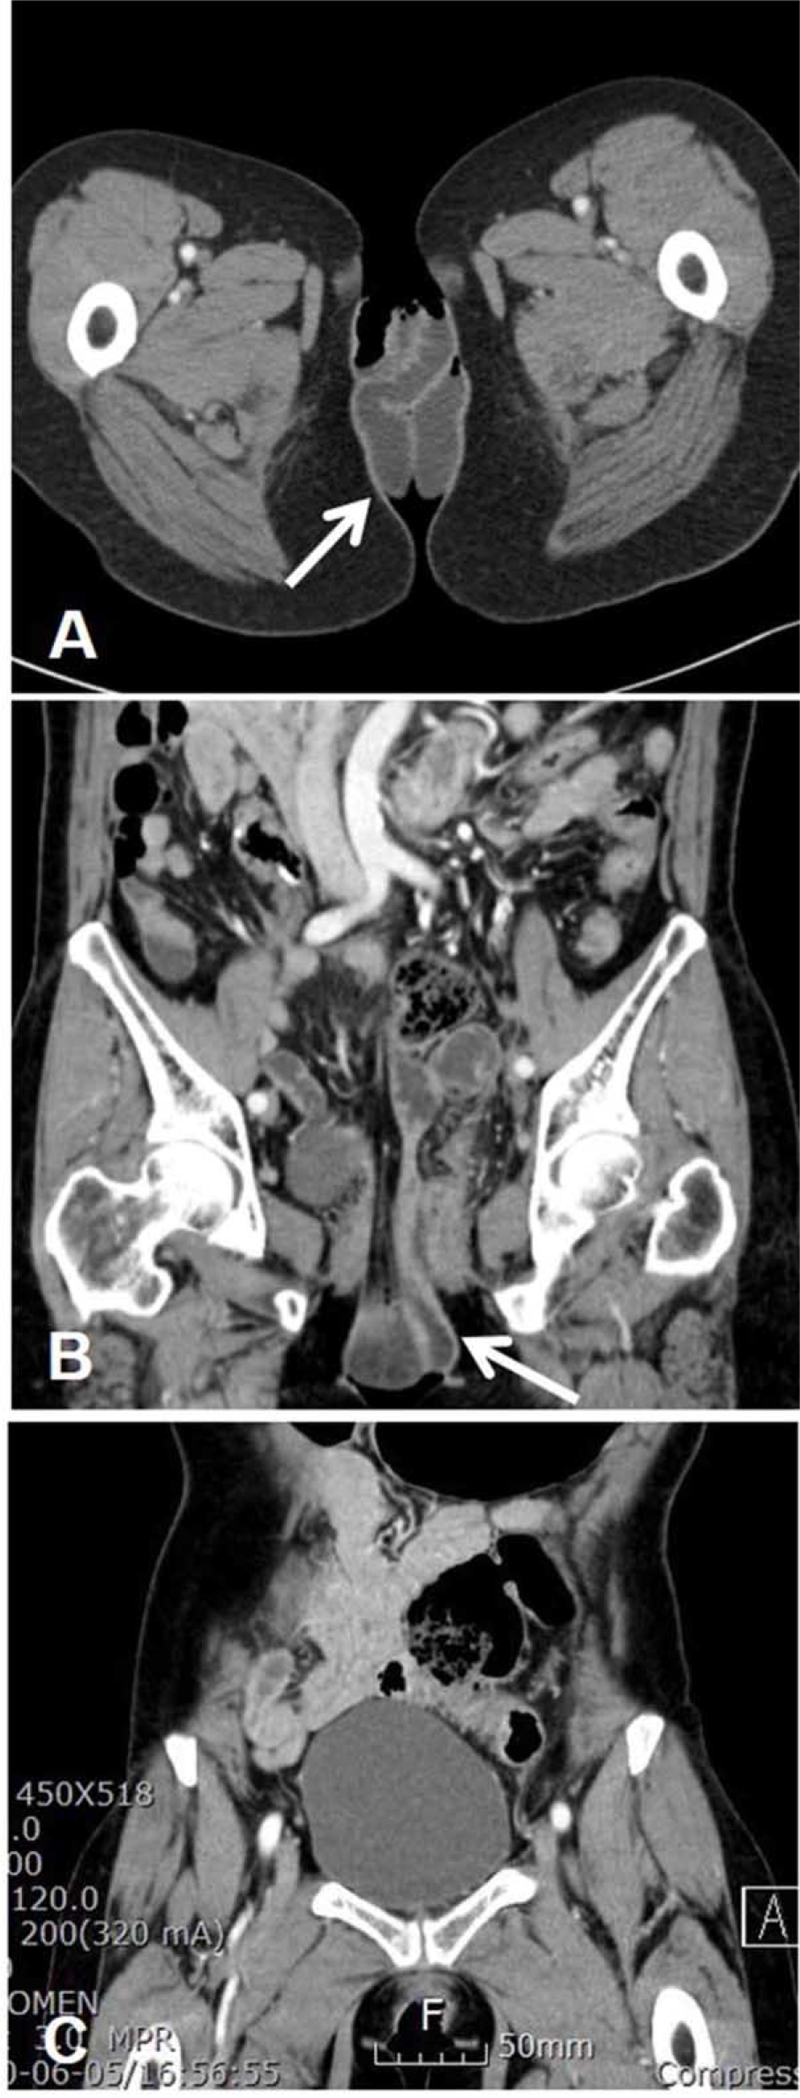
CT images of transvaginal evisceration of the small bowel. Axial (A) and coronal reformatted (B) contrast-enhanced CT images show herniation of small bowel loops (arrows) through the vaginal vault. (C) Distended bladder at presentation. CT = computed tomography.
